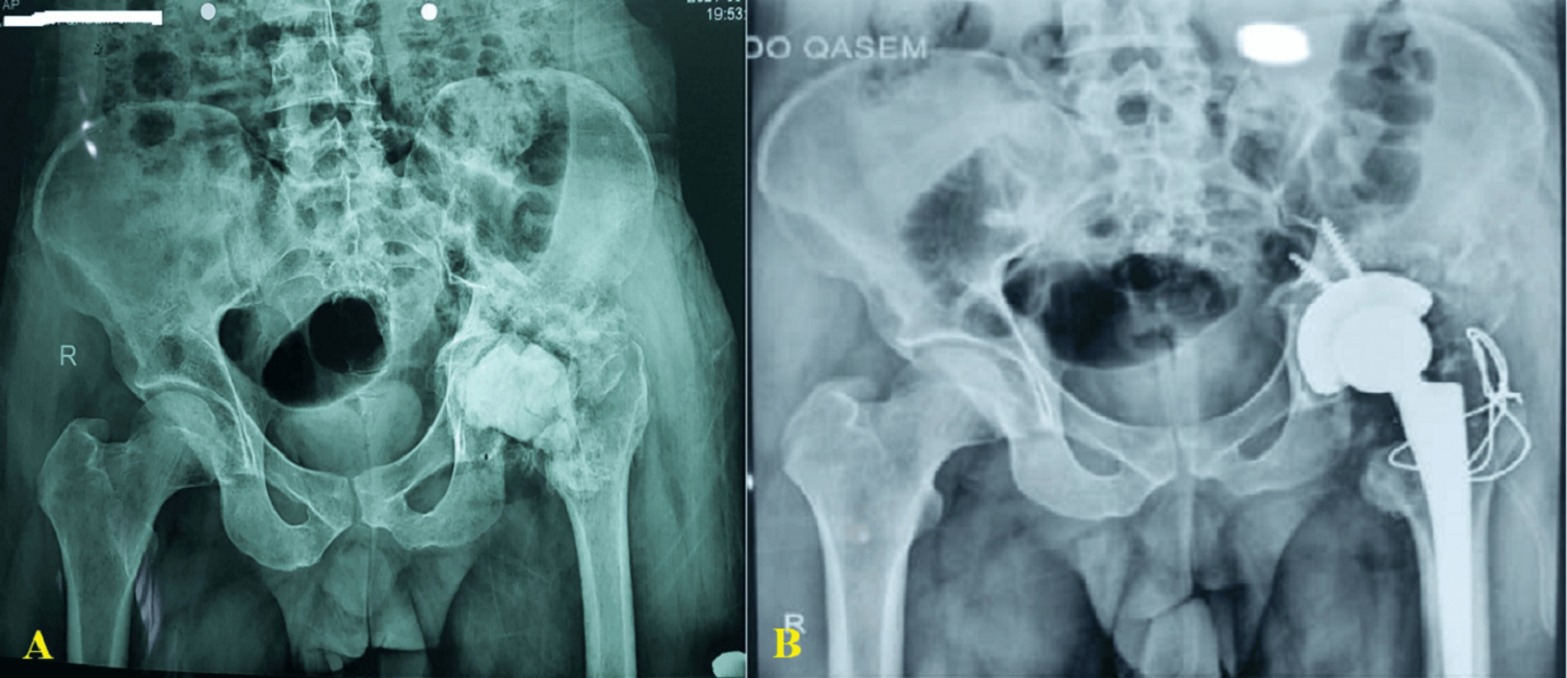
A radiologic X-ray shows (A) a 25-year-old male patient with a prior history of unsuccessful internal fixation and decreased abductor length following a gunshot wound; anteroposterior view. (B) Seven months after the accident, the patient underwent left femoral shortening osteotomy and cement-free total hip arthroplasty; anteroposterior view. Image credits: Abdulrakib Almirah, author
